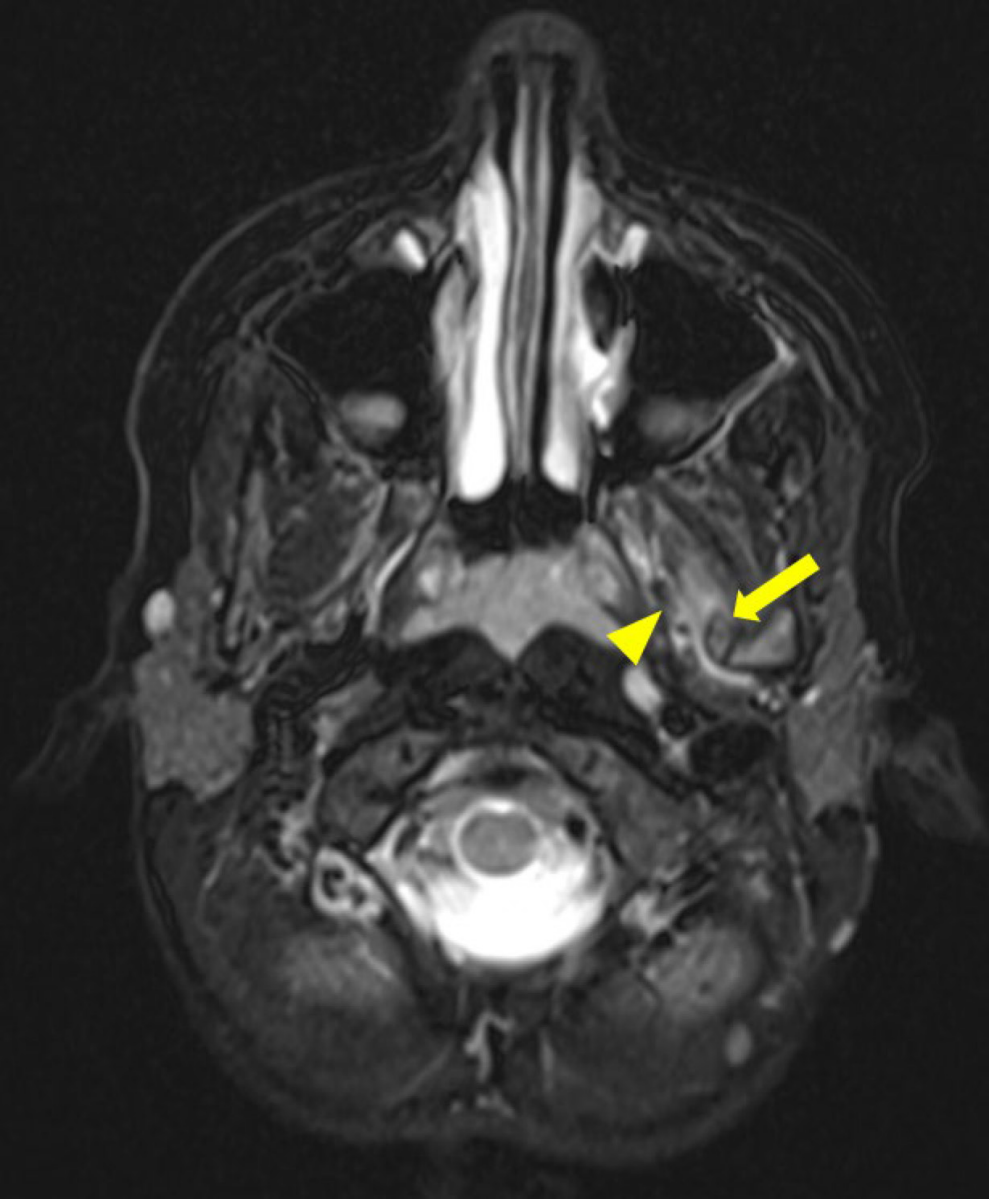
Axial T2 Dixon MRI scan showing edema infiltrating the lateral pterygoid muscles fibers (arrowhead) close to the ossified sphenomandibular ligament (arrow).
